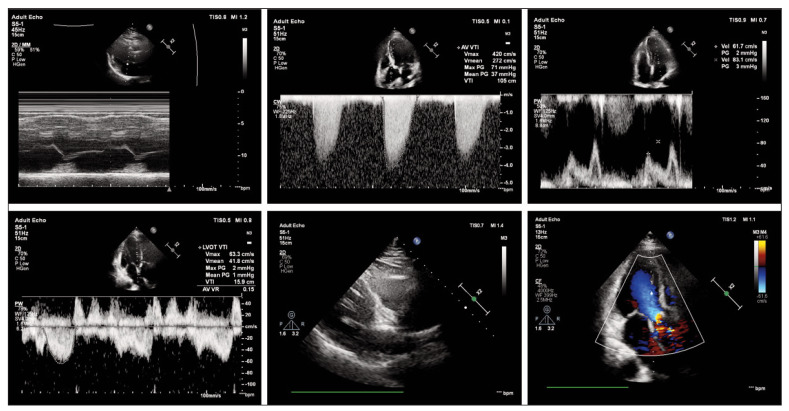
Echocardiographic findings of a patient. Female, 71 years old.
